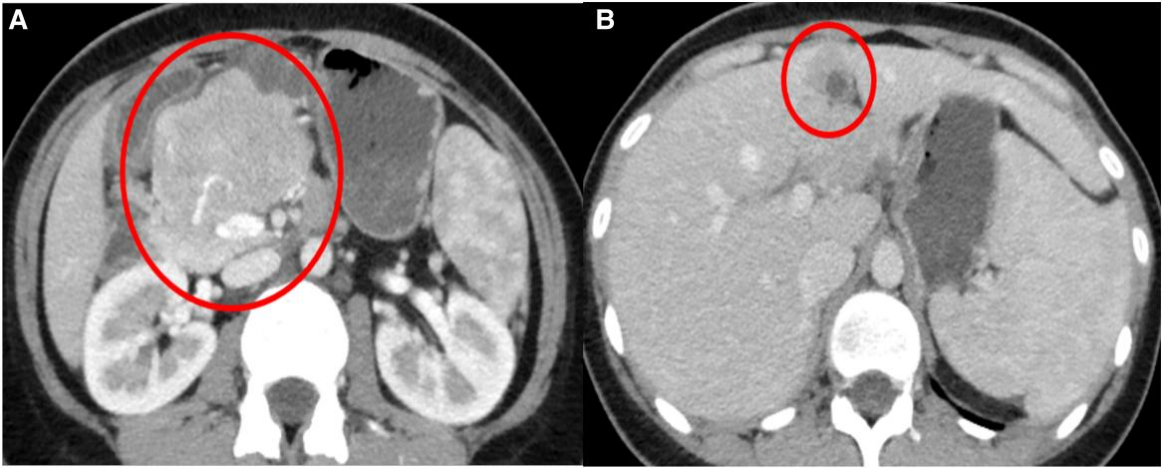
Contrast-enhanced axial computed tomography image of the abdomen showing a large, lobulated, enhancing soft-tissue mass arising from the pancreatic head-body with intralesional hyperdensities indicative of vascularity (A). An enhancing nodule with central fluid density at segment IVB of the liver suggestive of hepatic metastasis (B).
